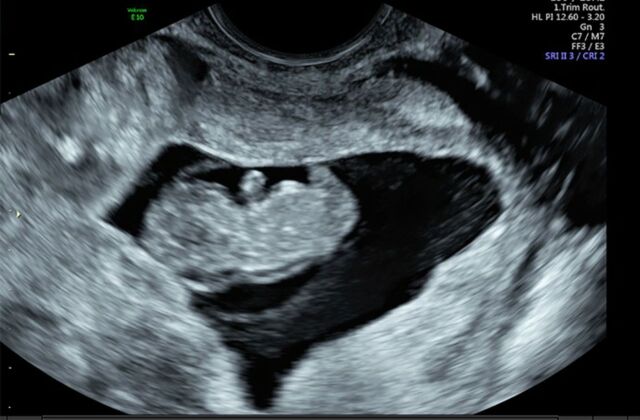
Ultrasound demonstrating fetal pole consistent with 13 weeks’ gestation.
